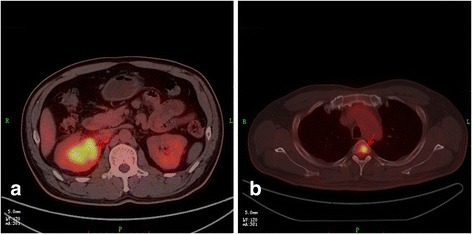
A positron emission tomography (PET) - computed tomography (CT) scan was made. Evidence of intense FDG uptake in irregular mass centered within the superior pole of the right kidney (a, arrow) well as diffuse osseous metastatic disease in third thoracic vertebra (b, arrow)
